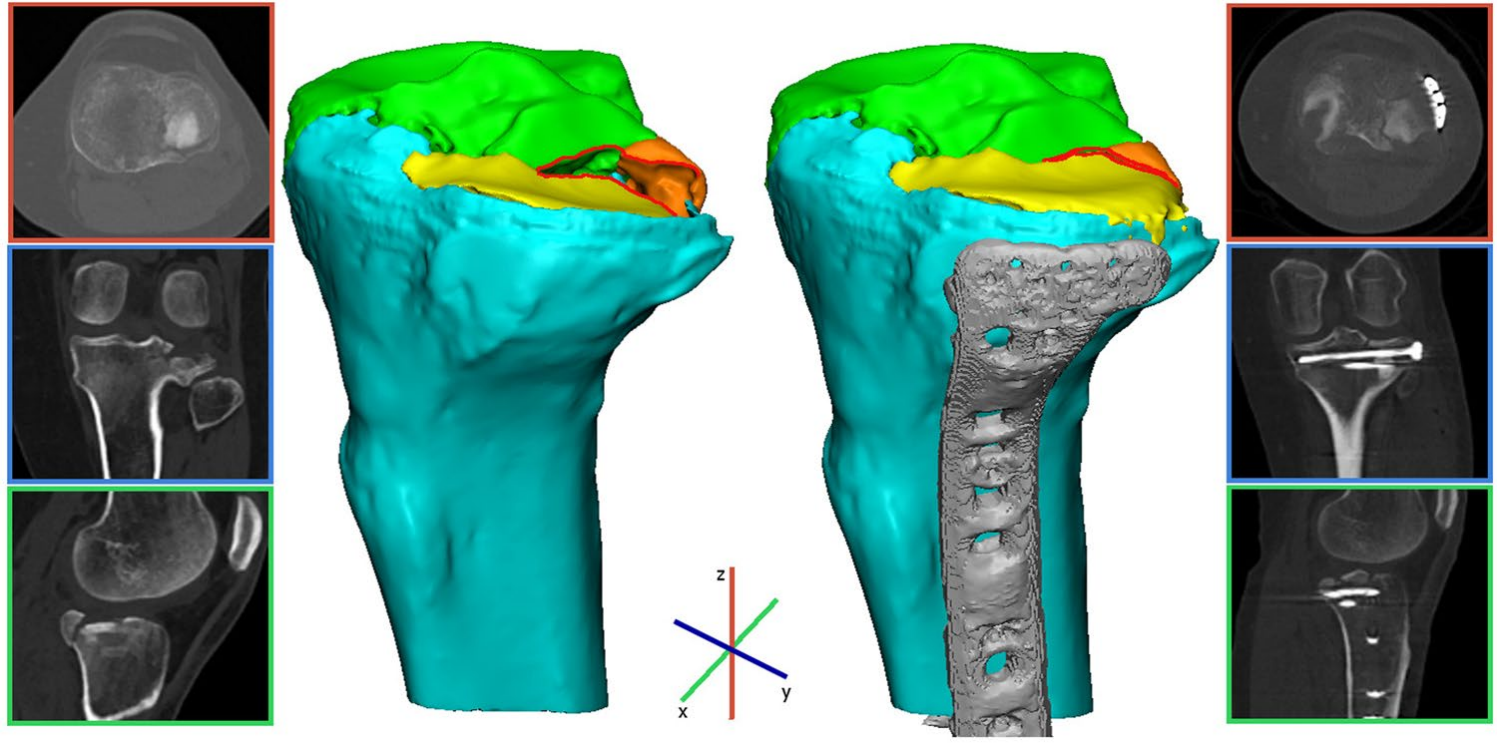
These images represent a clinical case in which we demonstrate the application of the quantitative 3D fracture measurements. Pre- and postoperative 3D assessment of a patient who was operated on a Schatzker 3 tibial plateau fracture. A 3D model of the pre-operative CT scan displayed a mean 3D gap of 0.5 mm, a step-off of 3.3 mm, a gap area of 27 mm^2^ with an articular surface involvement of 32.5% consisting of 3 fracture fragments. An open reduction and plate osteosynthesis was performed. A 3D model of the postoperative CT scan demonstrated an anatomical reduction of the fracture with a 20% decrease in the mean 3D gap (0.5 mm pre vs 0.4 mm postoperative), an 85% decrease in the mean 3D step-off (3.3 mm pre vs 0.5 mm postoperative), and a 10% decrease in the gap area (27 mm^2^ pre vs 24 mm^2^ postoperative).
